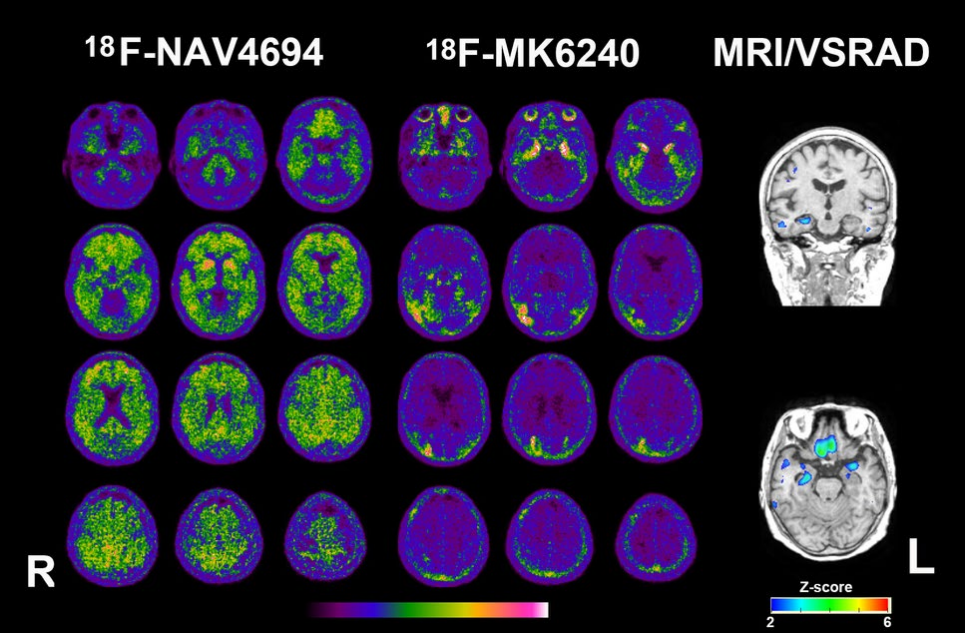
PET and MRI of a woman in her late 70s with AD (A+T+N+). Amyloid PET Centiloid scale is 86. Tau PET Braak stage is determined to be V, as tau deposition is observed in the bilateral medial temporal regions and the right temporoparietal cortex. The *Z*-score color map, analyzed using VSRAD software, demonstrates significant atrophy in the mesial temporal regions with a *Z*-score of 2 or higher
